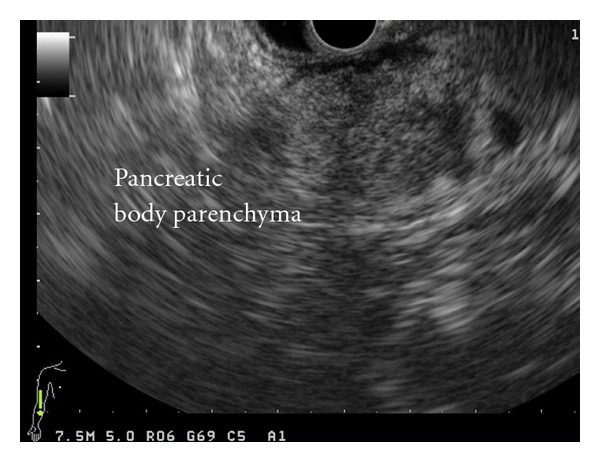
Linear EUS showing a markedly inhomogeneous pancreatic body parenchyma. The ultrasound wave was unable to delineate anatomical structures corresponding to either the main or the secondary pancreatic duct.
